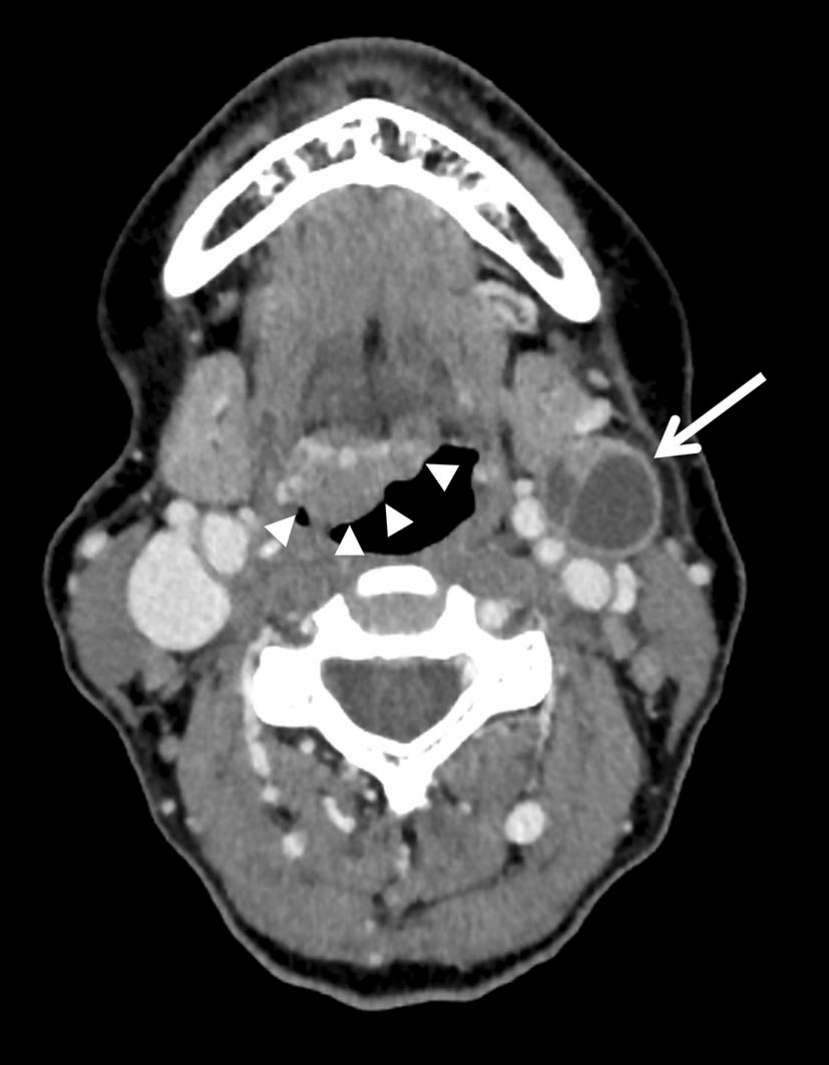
Axial CT slice showing a P16+ tumour of the base of tongue (arrowheads) and a typical cystic node without extra-nodal extension in the IIa left area (arrow) of a 53-year-old female with no history of tobacco or alcohol abuse.
